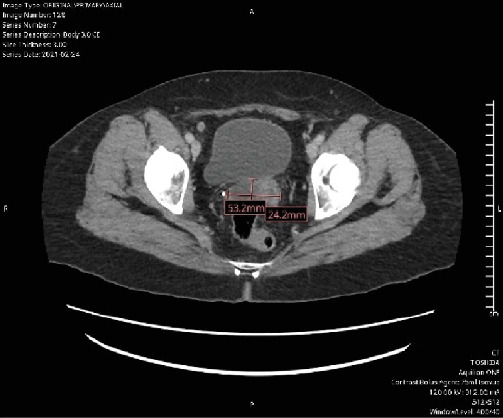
CT scan at time of recurrence. Axial image identifying 5.3 *cm* × 2.4 *cm* complex, low-density cystic lesion.
